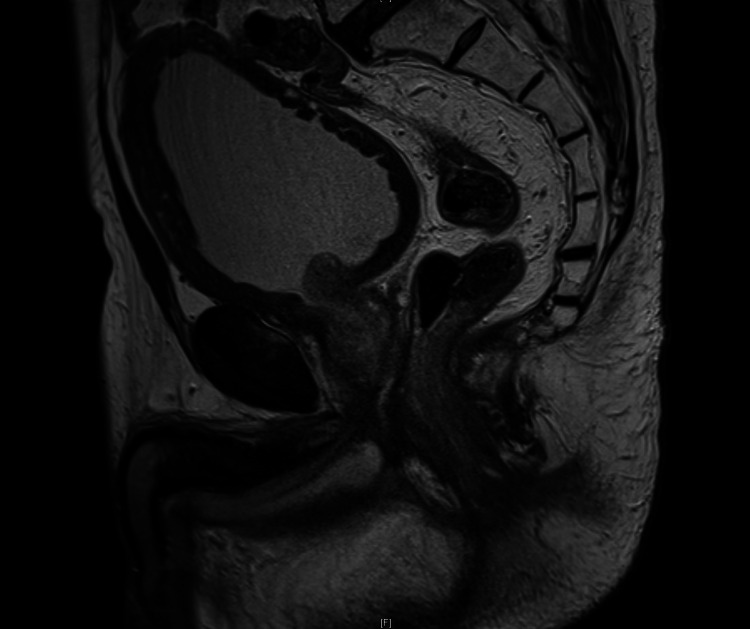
MRI Rectum Sagittal T2 section of an MRI rectum post-chemoradiation and prior to the APR. It demonstrates multiple small diverticula in the bladder wall and features of trabeculation.
